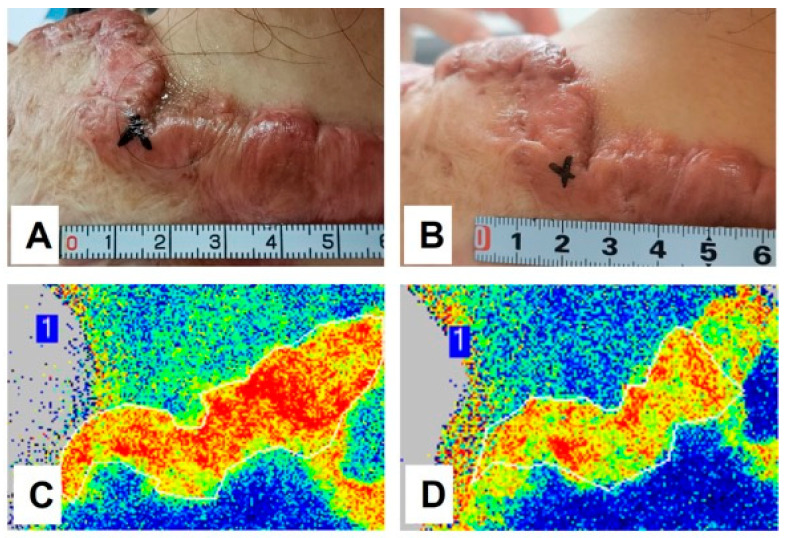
Case 5: A female patient presented with a large, long-standing keloid on her left shoulder, accompanied by marked erythema and symptoms of pain and itching (A). She received oral asiaticoside treatment for six months, which resulted in a notable reduction in scar thickness (from 5.68 mm to 4.68 mm measured by ultrasound) and erythema, accompanied by significant symptomatic improvement (B). The treatment also led to a substantial decrease in keloid vascularity, as measured by laser speckle contrast imaging (from 180 units, (C), to 153 units, (D)). Note, laser speckle contrast imaging (LSCI) is a powerful, non-invasive optical imaging technique used primarily to visualize and measure blood flow in tissue. The picture comes from the physician’s clinical database.
